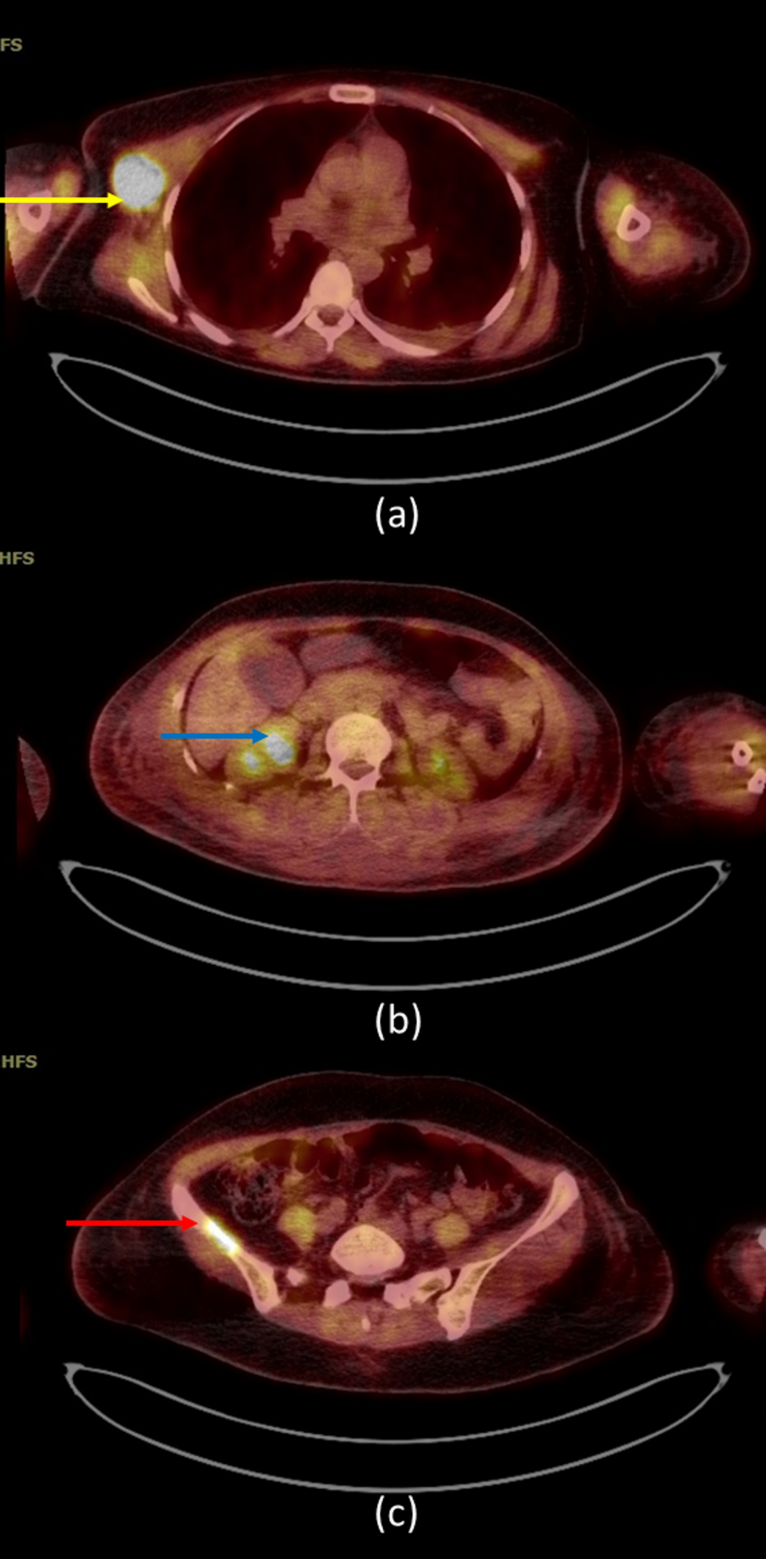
Positron emission tomography (PET) scan shows (a) two 18F-fluorodeoxyglucose (FDG) lesions at the upper outer quadrant of the right breast corresponding to soft tissue lesions (yellow arrow), with a maximum standardized uptake value (SUV)max of 13.38, compatible with focal right breast malignant disease; (b) FDG-avid right suprarenal lesion (blue arrow) with an SUVmax of 12.98, corresponding to right adrenal nodule, metastatic in nature; and (c) a large FDG-avid lytic lesion at the right iliac bone (red arrow) with an SUVmax of 7.92, also metastatic in nature.
